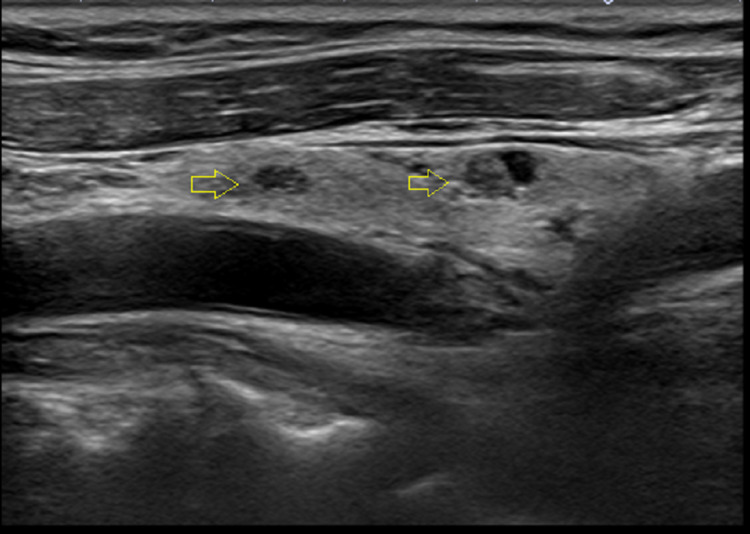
Thyroid ultrasound in Case 2: long axis showing small benign-appearing nodules in the right lobe
